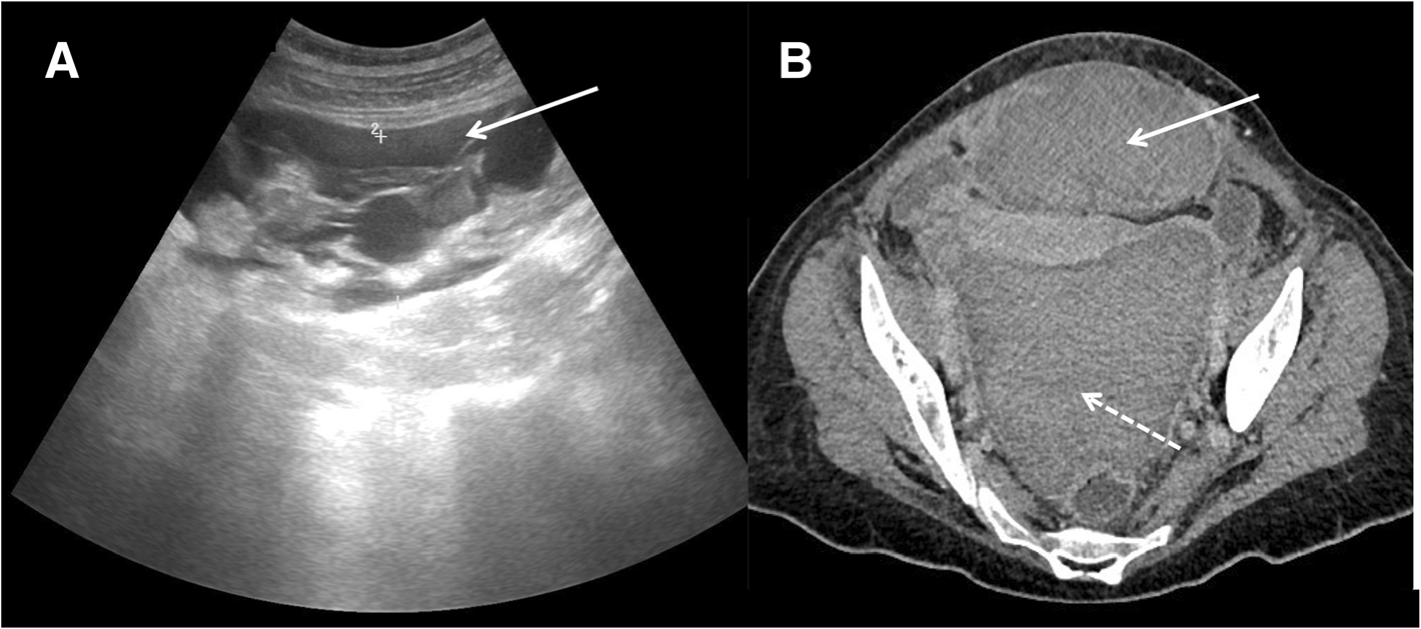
Subfascial hematoma associated with massive hemoperitoneum. a US examination showed a complex collection in this case in the rectus muscles (white arrow). b After contrast CT imaging confirmed the subfascial hematoma (white arrow) and showed also hemoperitoneum (dashed arrow)
